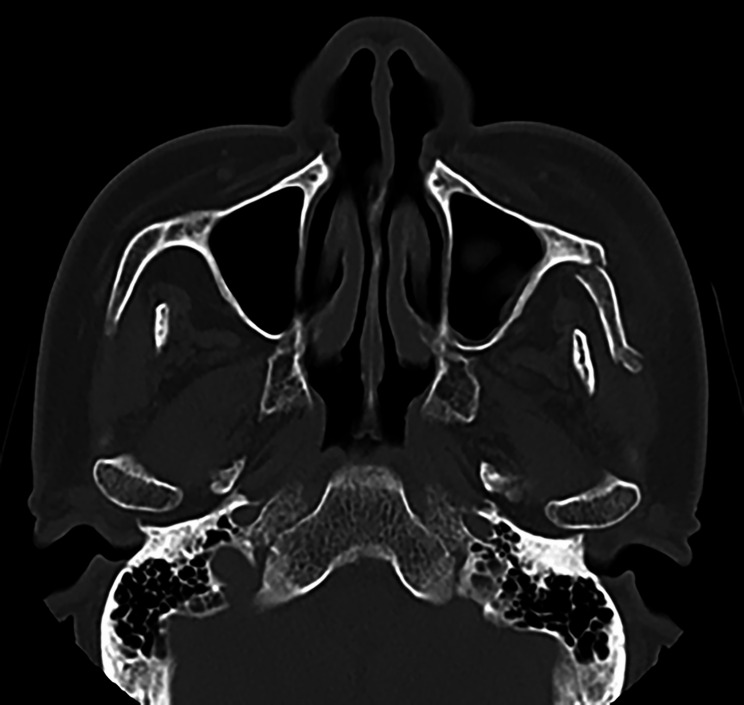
CT image at initial examination. The left zygomatic maxillary suture was fractured, and zygomatic arch was depressed. Zygomatic bone was displaced medially and interfered with the left muscular process
